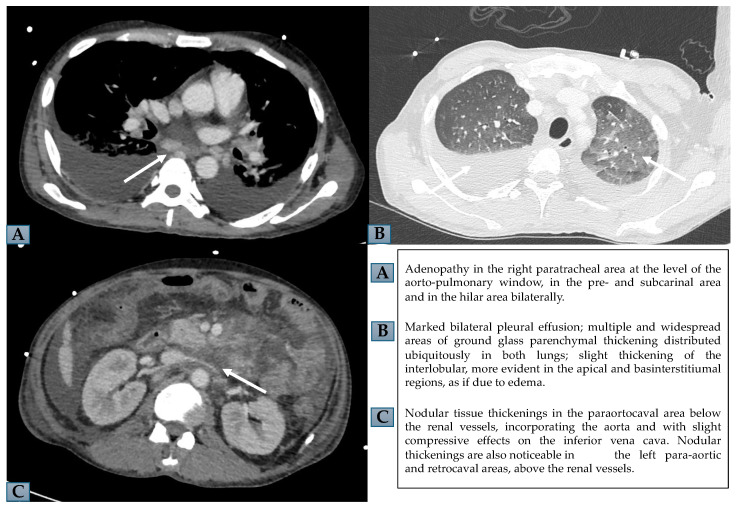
Chest and abdomen CT. (A,B) show mediastinal adenopathy and bilateral pleural effusion with ground glass parenchymal thickening, in chest CT. (C) displays nodular thickenings in paraortocaval and retrocaval areas, in abdomen CT.
